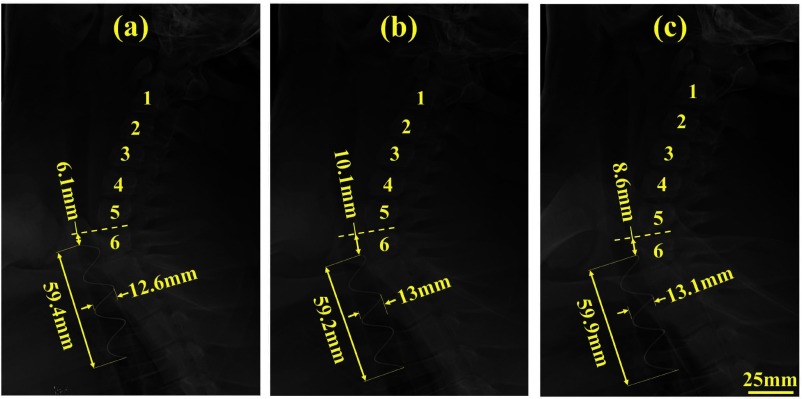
Lateral view x-ray images taken on (a) day of stent deployment, (b) day 7 after stent deployment, and (c) day 21 after stent deployment. Numbers 1–6 indicate vertebrae. Measurements indicate displacement with respect to 6th vertebra as well as diameter and length of stent.
